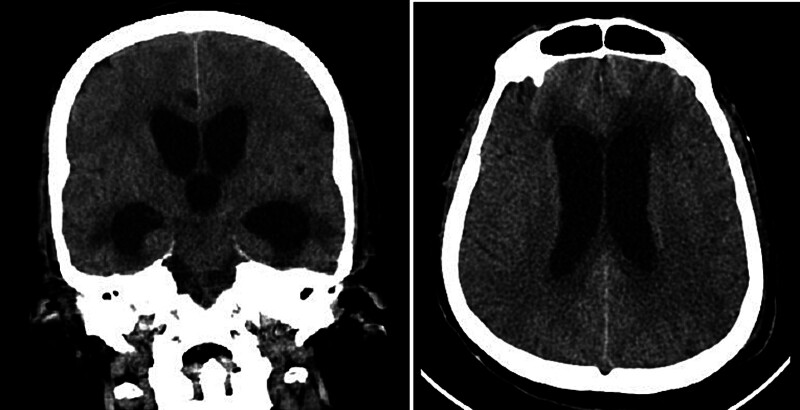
CT imaging of the brain showed hydrocephalus.
